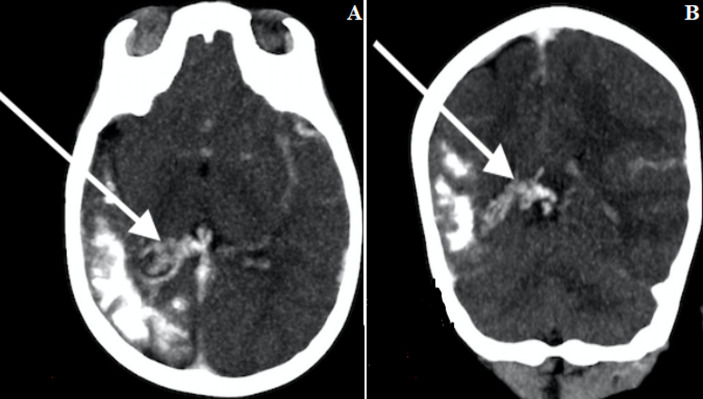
cerebral contrast CT in MIP A) (axial); B) (coronal) showing the right pial angioma in the form of an enlargement and dilation of the choroid vessels (white arrows)
